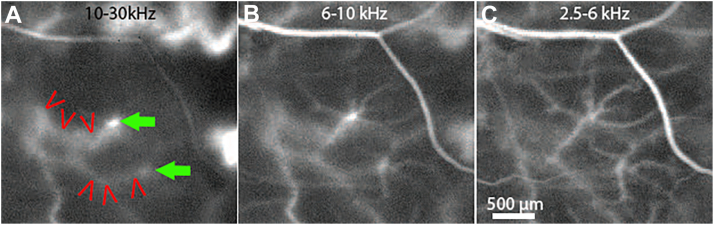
Characterization of precapillary complexes by stepwise Doppler shift thresholding. A, Laser Doppler holography imaging of second-order arterioles (red arrowheads) temporal to the macula, giving rise to 2 axial arteries (green arrows). B and C show 2 adjacent precapillary complexes.
